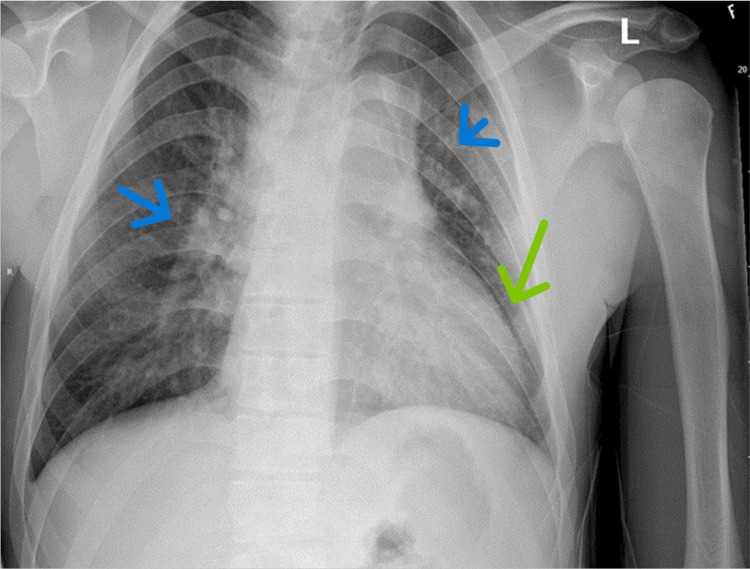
Chest radiograph (CXR) revealing an enlarged cardiomediastinal silhouette (green arrow), and well-inflated lung fields with faint airspace consolidations in the bilateral hilar regions (blue arrows). No pneumothorax was appreciated
